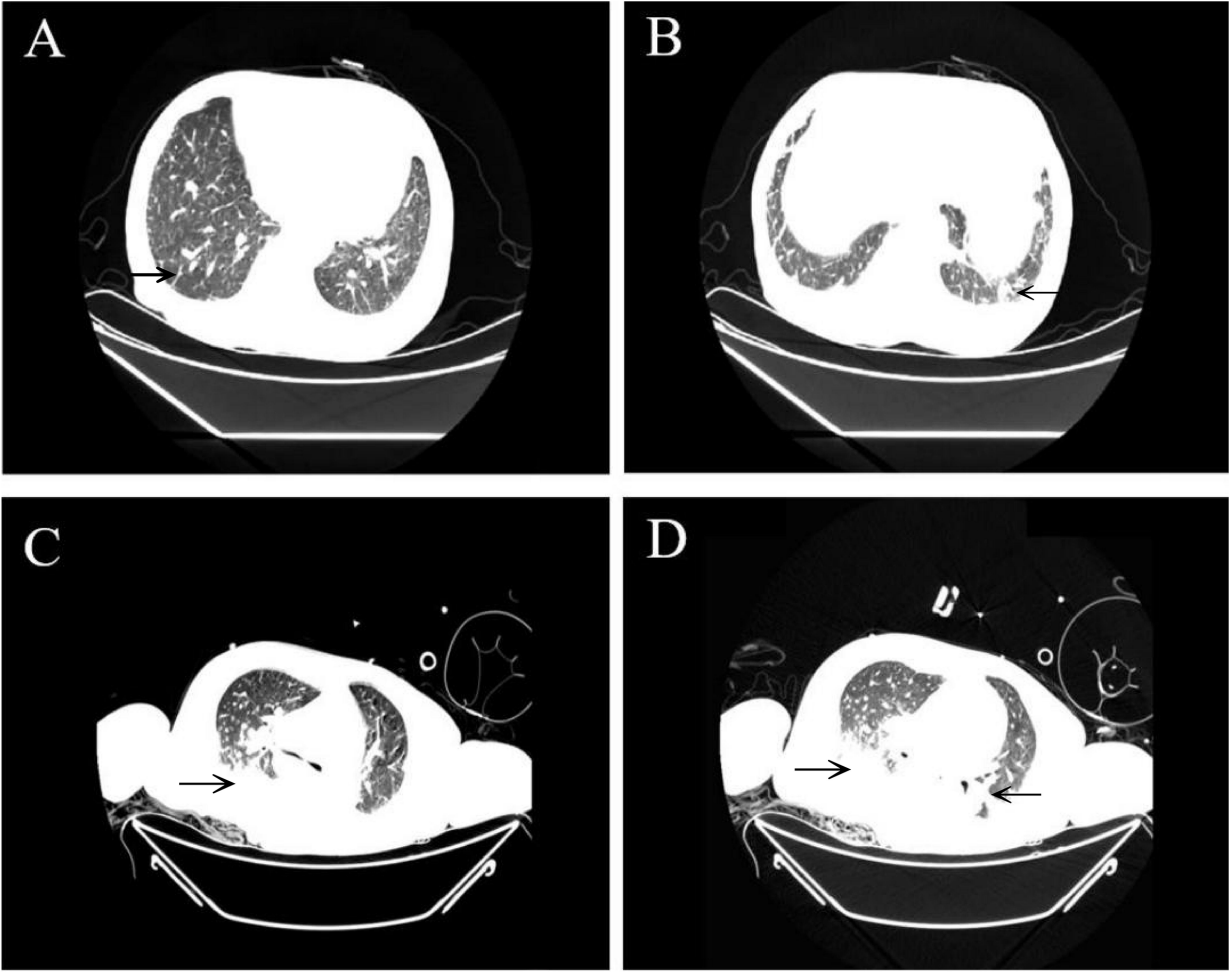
Chest CT scans during hospitalization and follow-up. Axial chest CT images obtained on the first (A, B) and sixth (C, D) days after admission. Black arrows depict progressive bilateral lower-lobe infiltrates and increasing pleural effusion.
